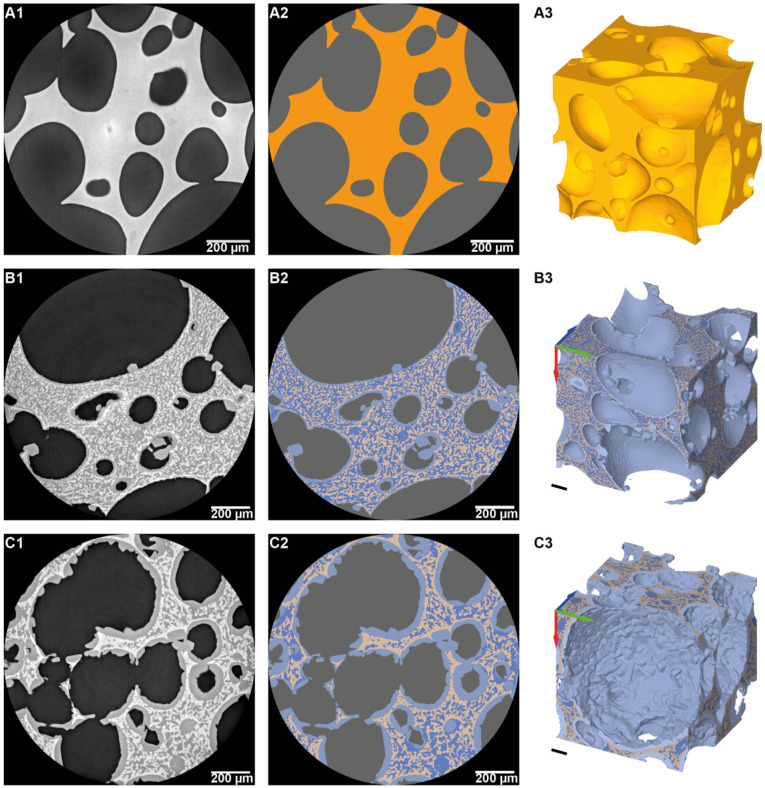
Synchrotron X-ray horizontal slices (grey level images in the left and segmented images in the right) and 3D visualization of the sample after segmentation: (A1–A3) Unfrozen sponge cake; (B1–B3) Model sponge cake sample frozen at −17.2 °C min^−1^ (fast freezing); (C1–C3) Model sponge cake sample frozen at −0.3 °C min^−1^ (slow freezing). (Air in grey, ice outside the matrix in light blue, ice inside the matrix in dark blue and starch in brown). Each cube is 1 mm a side.
